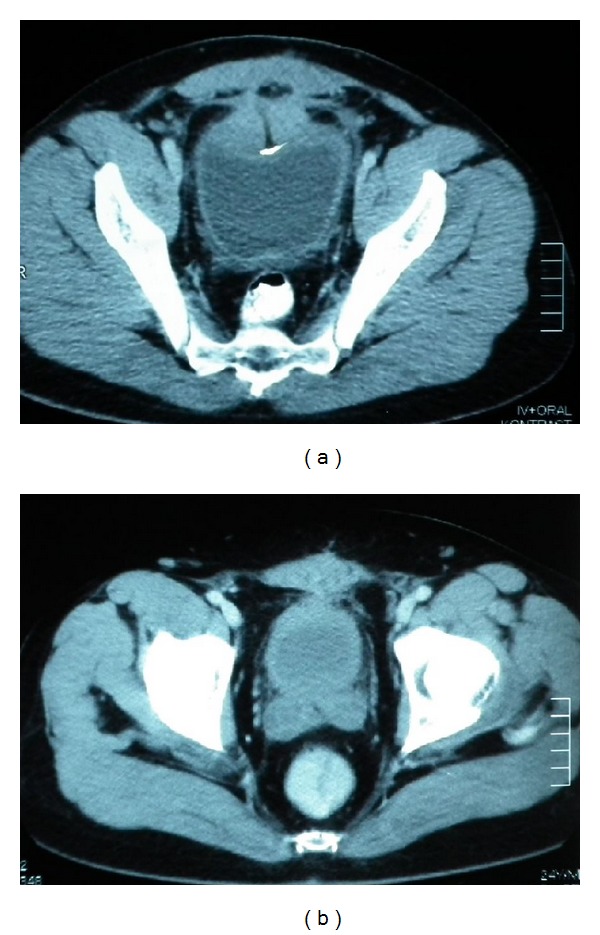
(a) A computed tomography (CT) scan of the abdomen and pelvis showing a mass lesion, involving the dome of the urinary bladder, invading the rectus fascia and muscles. (b) CT scan of the abdomen and pelvis showing no evidence of the mass at the postoperative 6th month.
